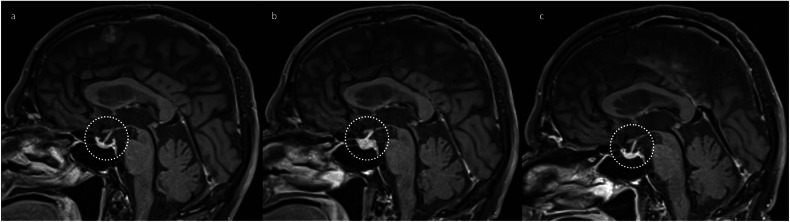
Serial sagittal post-contrast T1-weighted images of the pituitary gland (dotted circle), demonstrating the typical temporal evolution of hypophysitis (which developed after two cycles of combination ipilimumab and nivolumab for metastatic melanoma): enlargement (b) compared to baseline (a), followed by a decrease in size (c)
